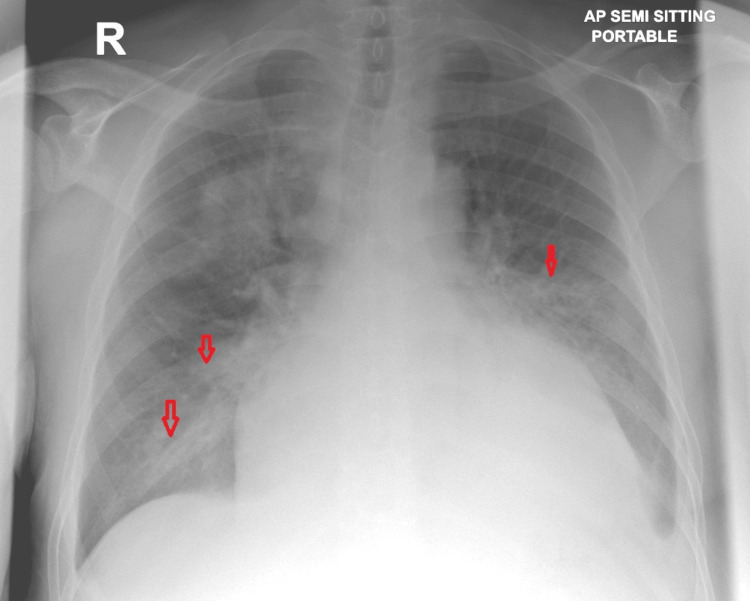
Case 2: Chest X-ray The chest X-ray reveals an enlarged heart, bilateral pleural effusions, and bilateral perihilar and mid-to-lower zone lung consolidation.
